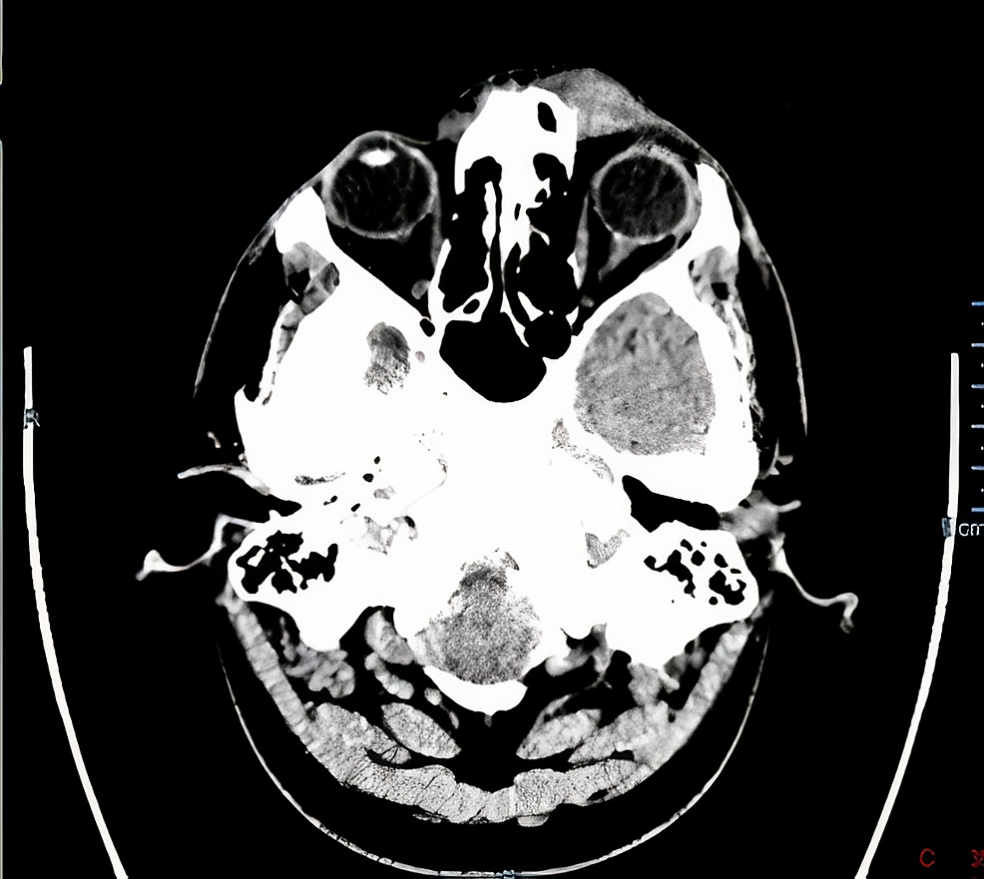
Computed tomography (CT) of the head with contrast showing a well-defined hyperdense subcutaneous soft tissue lesion seen at the medial aspect of the left orbit (eyelid-extra orbital).
